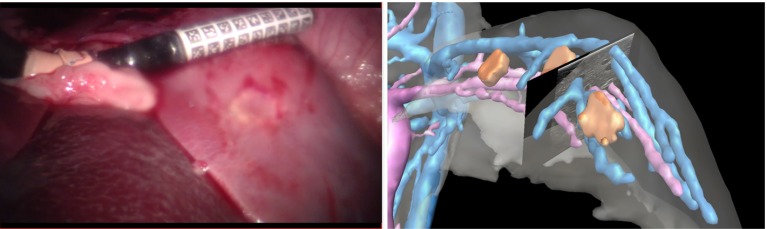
Applying the registration transformation to anatomical models derived from preoperative CT data enables live visualisation of CT data, within the context of live laparoscopic video and ultrasound data
